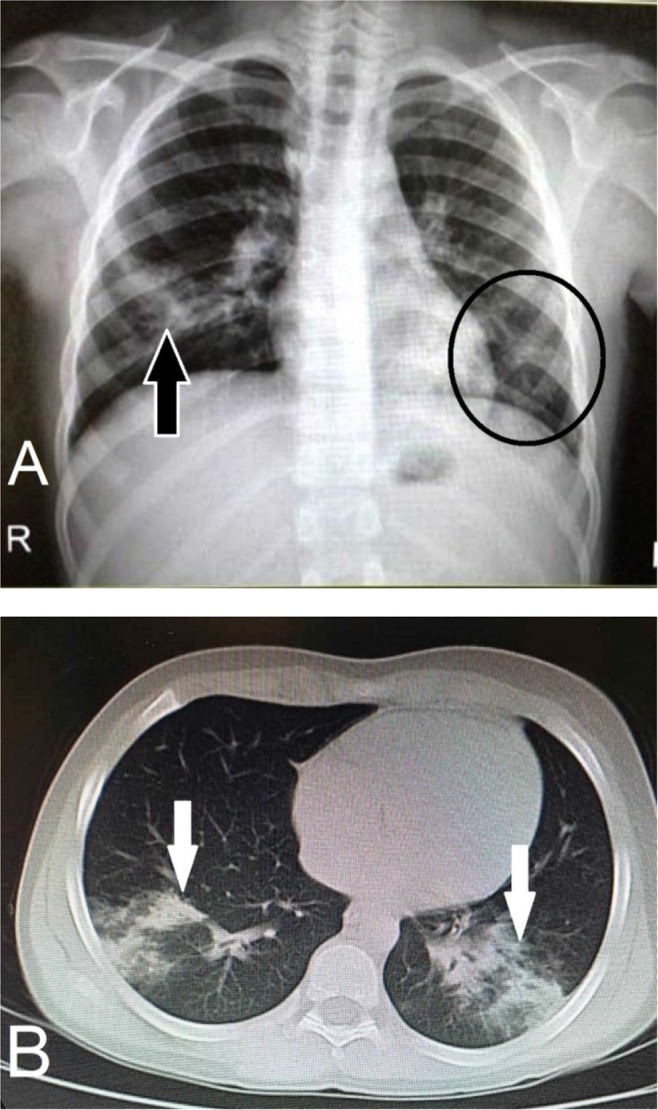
A) In chest X-ray radiography, air space opacification is visible in the right lower lobe (black arrow), and faint ground-glass opacity can be seen in the left lower lobe (encircled). B) The axial view of chest CT scan for the lungs shows patchy lower lobe consolidaions bilaterally (white arrows).
